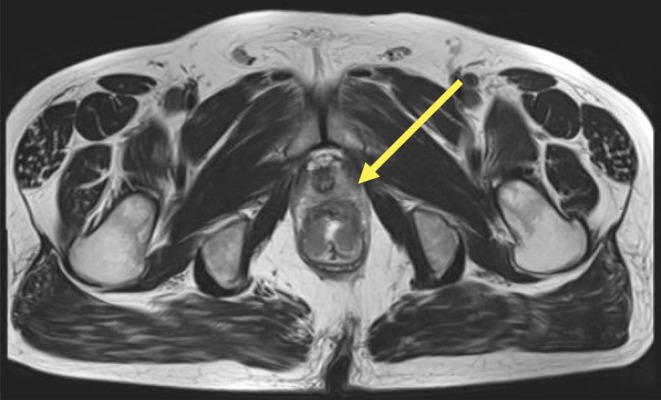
Preoperative MRI showing suspected extramural invasion of the anterior rectal wall (yellow arrow).
